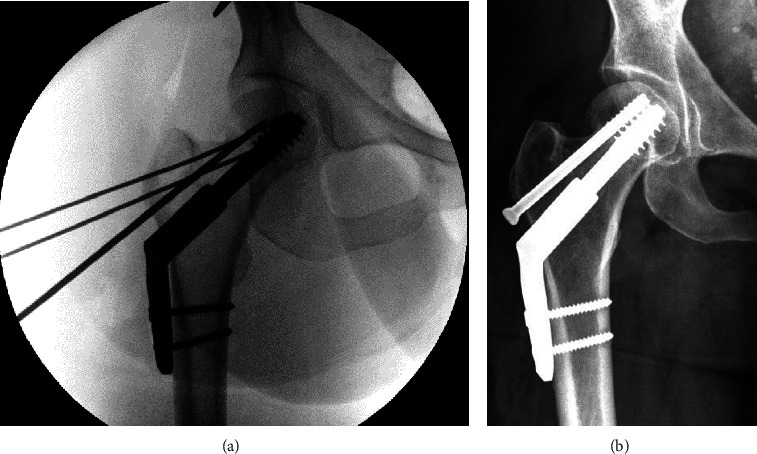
(a) Intraoperative right hip AP fluoroscopic image demonstrating reduction and implant placement with provisional fixation still in place; (b) final AP radiograph of the right hip demonstrating healed subcapital femoral neck fracture at 6 months postoperatively.
